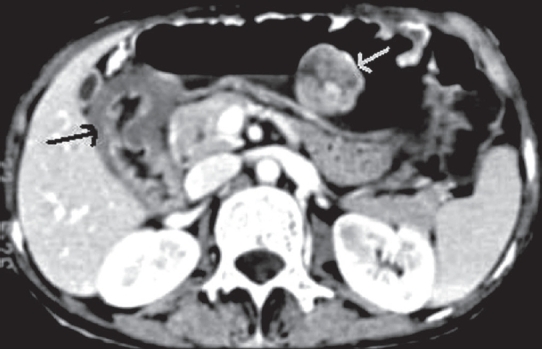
Contrast-enhanced axial CT image showing a neurofibroma along the greater curvature of the stomach along with diffuse thickening of the gastric outlet due to diffuse neurofibromatosis
